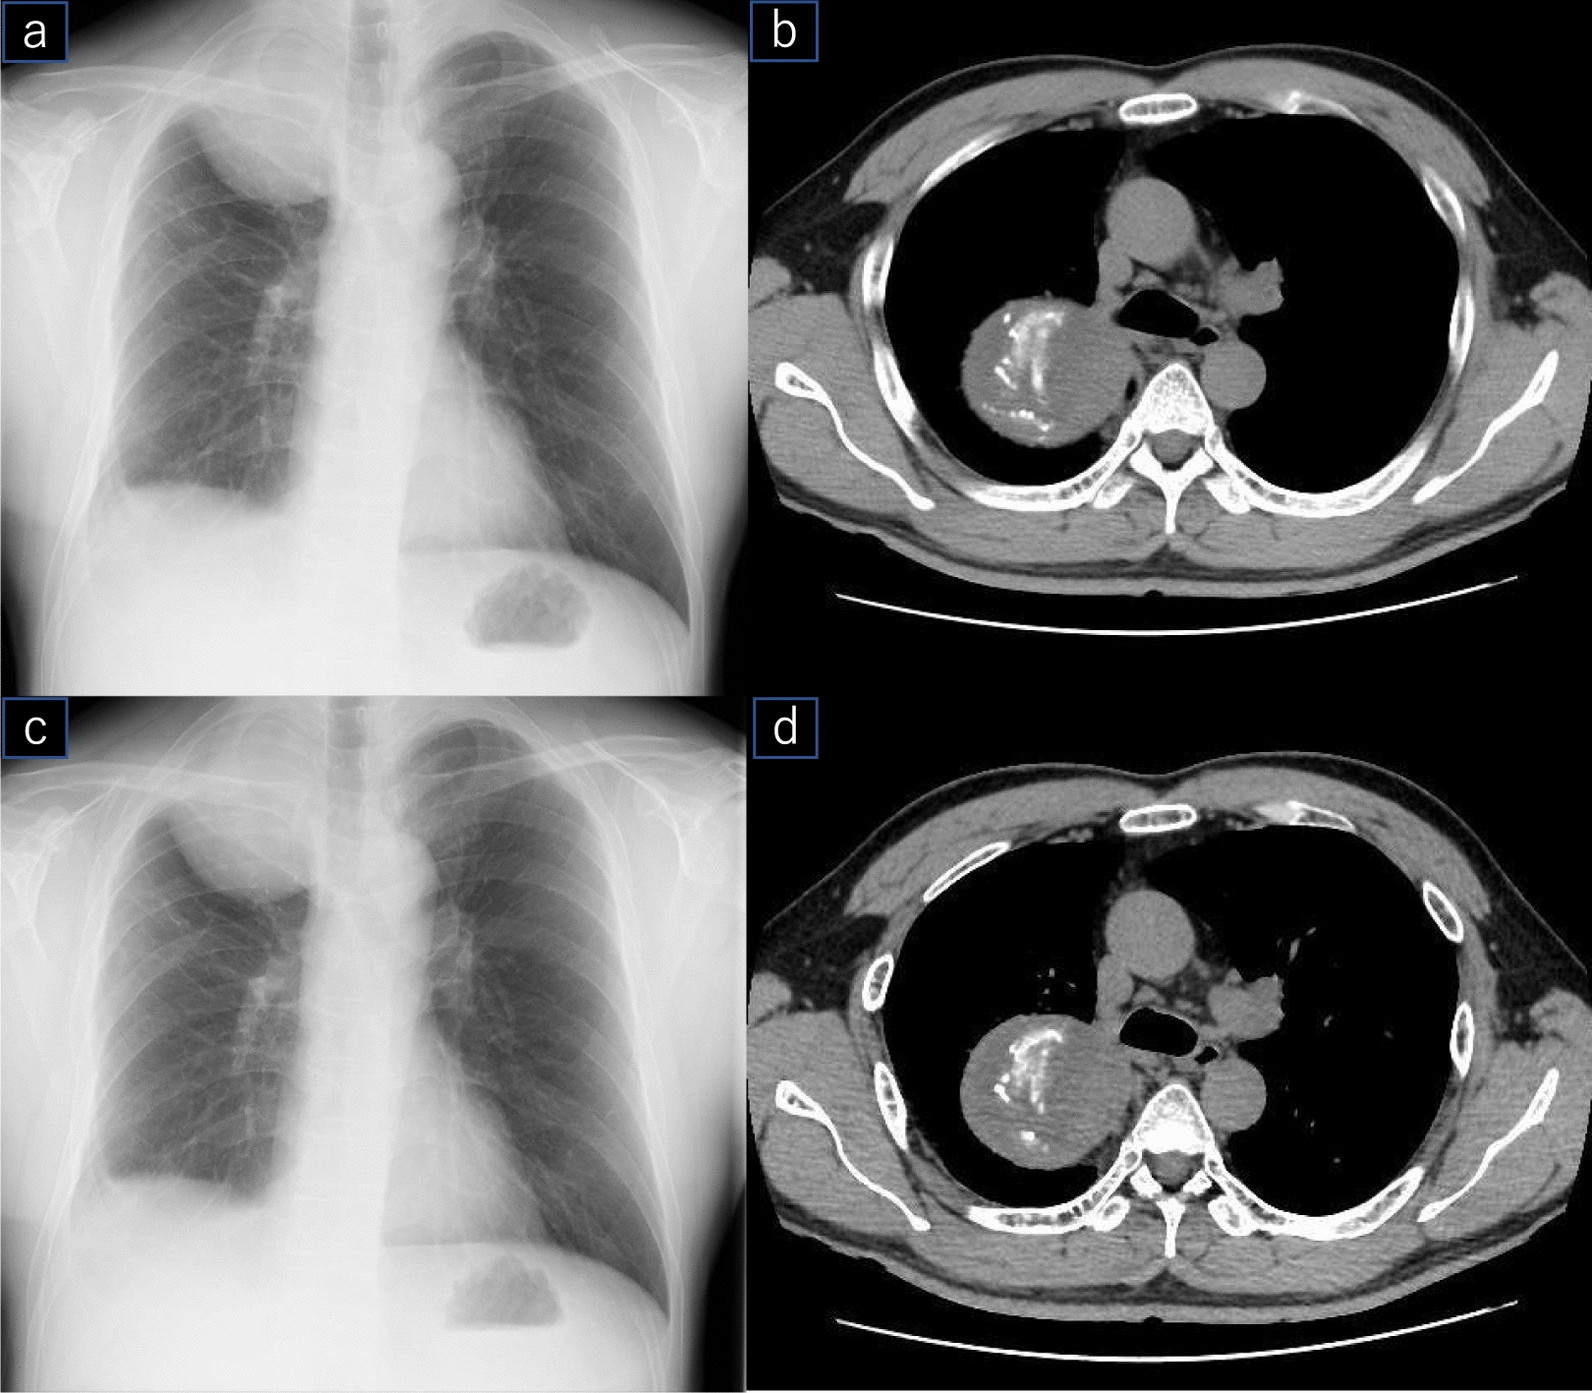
a On radiography, gauze fragments are not found in the mass. b Computed tomography reveals a gauze fragment with calcification in the granuloma. c Two years later, the mass has increased in size. d Granuloma is pressing on the trachea
